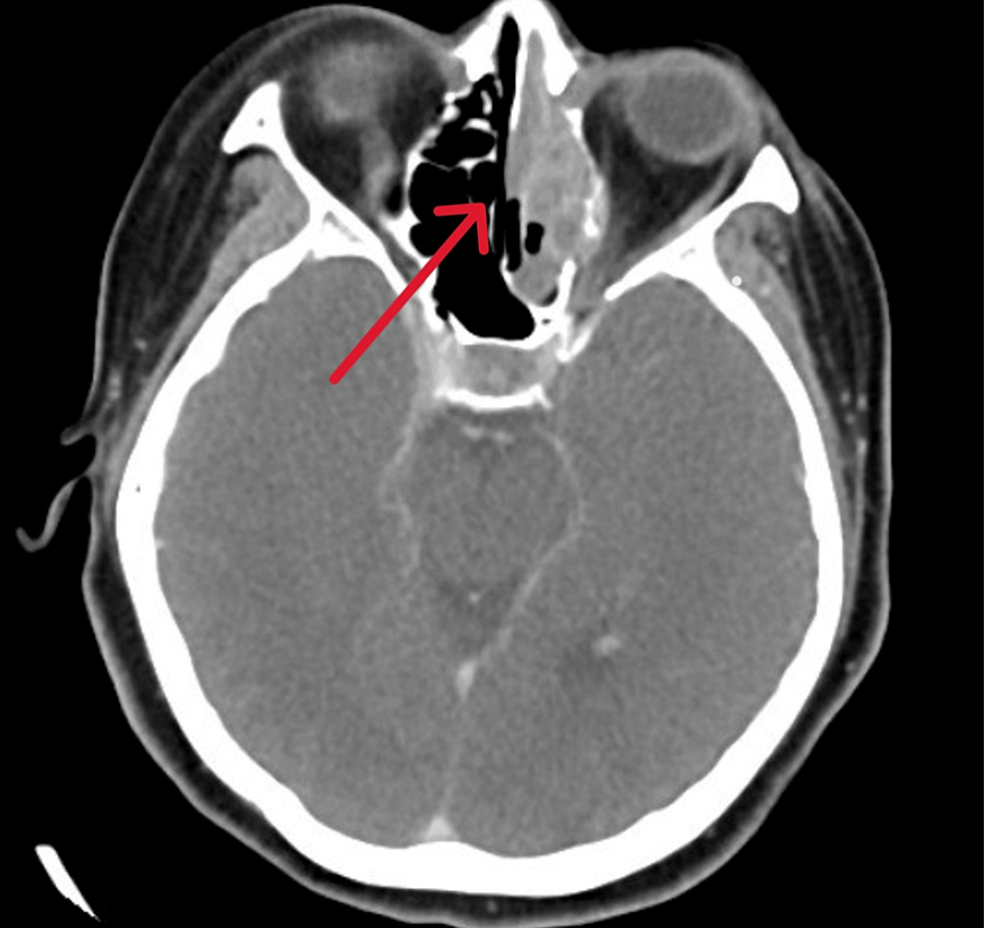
Case 1: CT axial view image shows a mass with its epicenter at the left anterior ethmoid (arrow)
